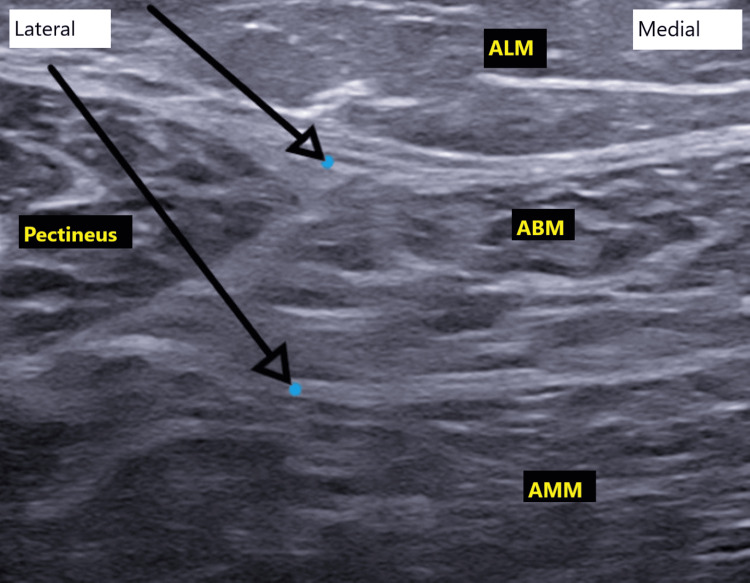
Ultrasound-guided obturator nerve block Ultrasound image showing the target points (blue dots) for final needle placement for local anesthetic injection between two muscle layers. ALM, adductor longus muscle; ABM, adductor brevis muscle; AMM, adductor magnus muscle; Pectineus: pectineus muscle.
